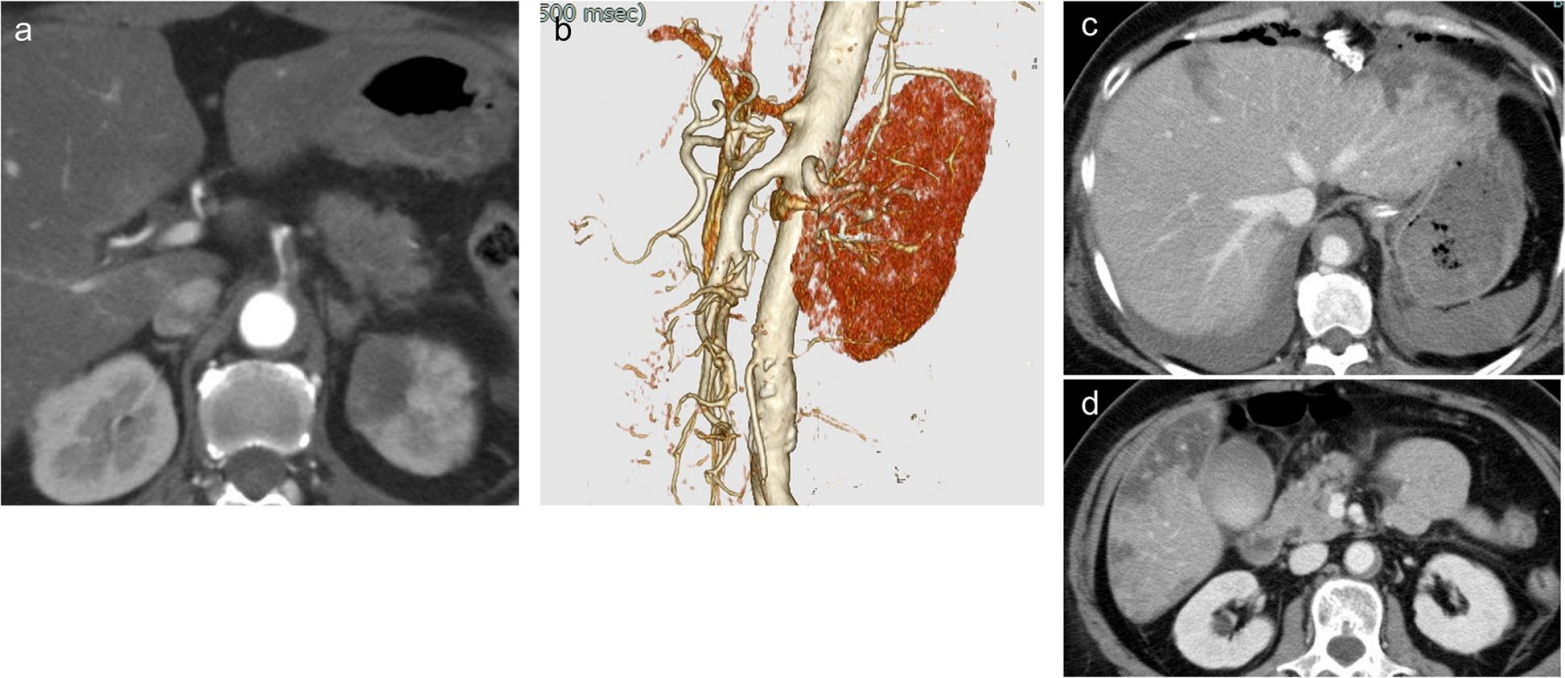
a, b, c, and d Postoperative contrast-enhanced CT. a and b Restenosis of the celiac artery. c and d An unenhanced liver image on CT
